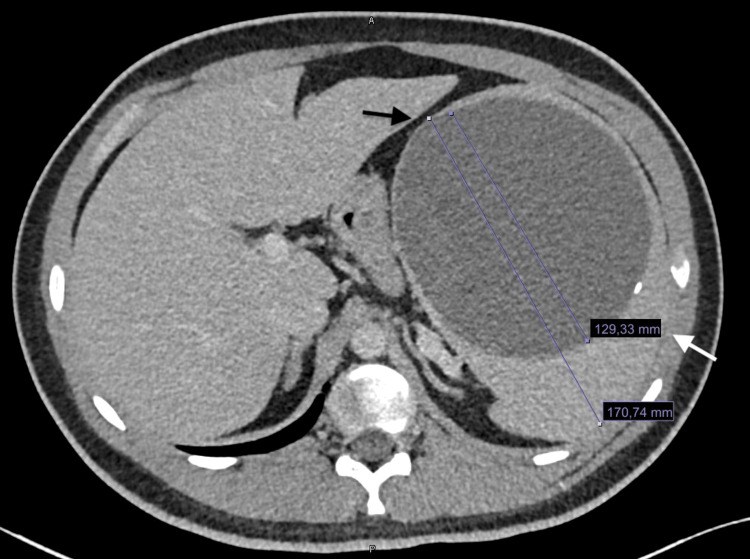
Contrast-enhanced computed tomography (axial view). Splenic measurement of 170.74 mm (black arrow). Splenic cyst measurement of 129.33 mm (white arrow).
